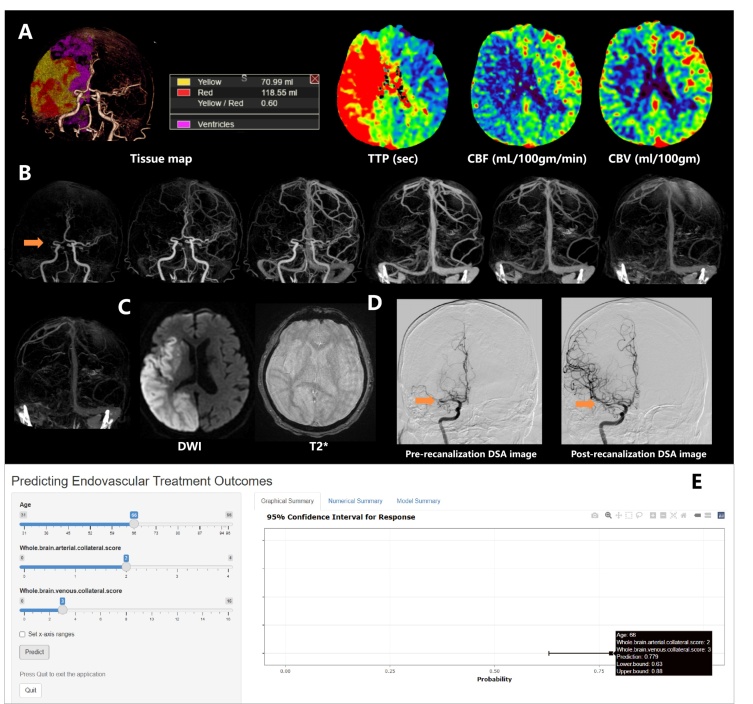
Case of futile recanalization and probability estimation. A 66-year-old patient presented with left-sided limb weakness and slurred speech for 8 hours. Medical history included coronary artery disease. The NIHSS score was 4. (A-B) CTA revealed right MCA occlusion, and CTP showed prolonged TTP with reduced CBF and localized CBV reduction. The whole-brain collateral scores were 2 for arterial and 3 for venous. (C-D) After EVT, the mTICI score was 3. At 90-day follow-up, the mRS score was 4, indicating an unfavorable outcome and futile recanalization. (E) The dynamic prediction system assessed the risk of futile recanalization for this patient. Clinical parameters entered were: age 66, whole-brain arterial collateral score of 2, and venous collateral score of 3. The system predicted a 77.90% probability of futile recanalization, consistent with the poor outcome.
